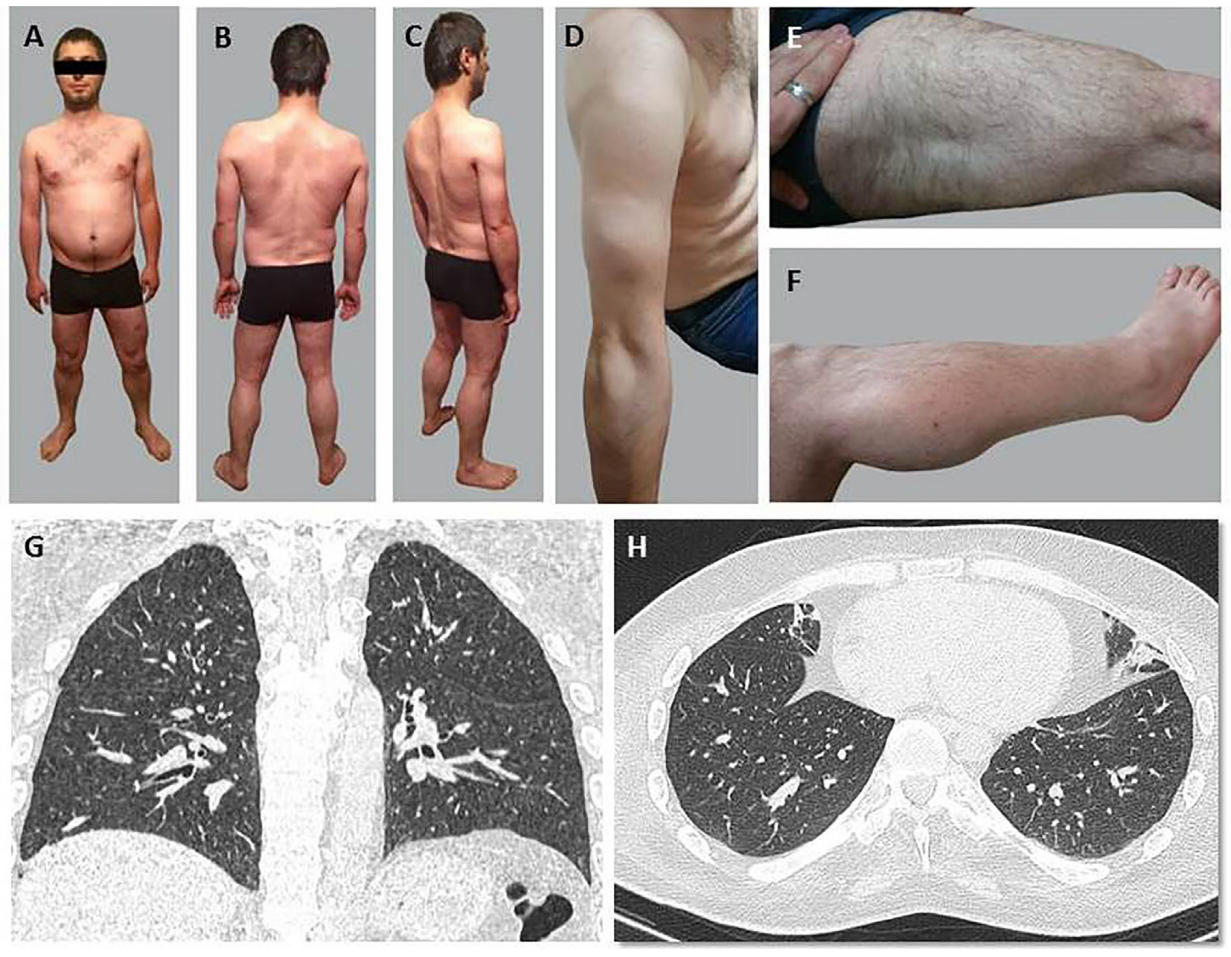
General appearance (A–F) and chest CT scans at the Th10-11 level (G,H) of Patient 1. (A–C) Evident amyotrophia in paravertebral muscles primarily of the thoracic spine. (D) Moderate amyotrophias in muscles of the shoulder girdle and proximal regions of the upper extremities. (E,F) Moderate pseudohypertrophy of deltoid and triceps muscles of the arm, quadriceps muscles of the thigh, and the gastrocnemius muscles. (G,H) A reticulo-nodular pattern in the form of slightly outlined centrilobular foci and subsegmental atelectasis in the basal areas of both lungs.
